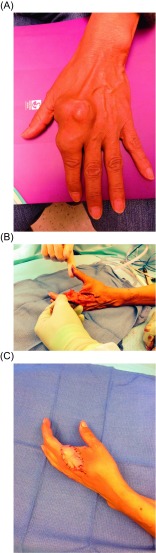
(A) Posterior-anterior (PA) and (B) oblique views of radiograph demonstrating chondrosarcoma at the thumb proximal phalanx. (C) PA and (B) oblique views postoperatively after resection and limb salvage surgery (LSS) reconstruction.
